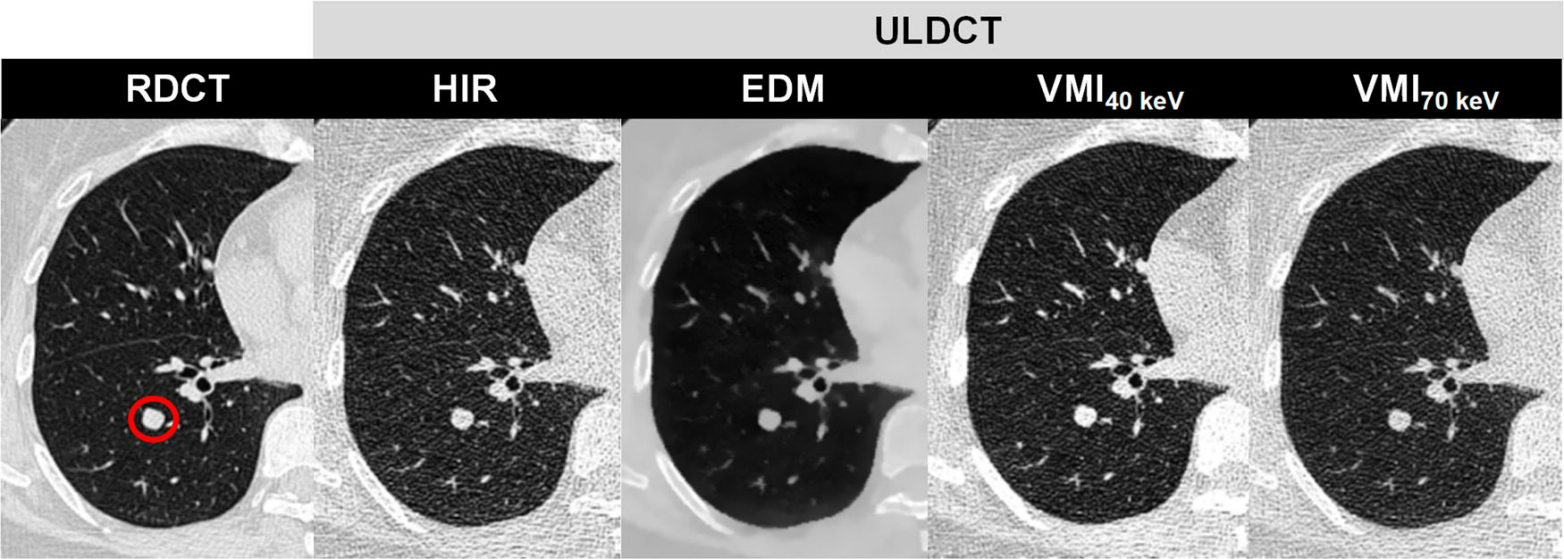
DLSCT at different radiation doses and reconstruction algorithms in a 65-year-old female (BMI: 21.2 kg/m^2^) with a solid nodule in the right lower lobe (circle). RDCT, regular-dose CT; ULDCT, ultra-low dose CT; HIR, hybrid iterative reconstruction; VMI, virtual monoenergetic image; EDM, electron density mapping
